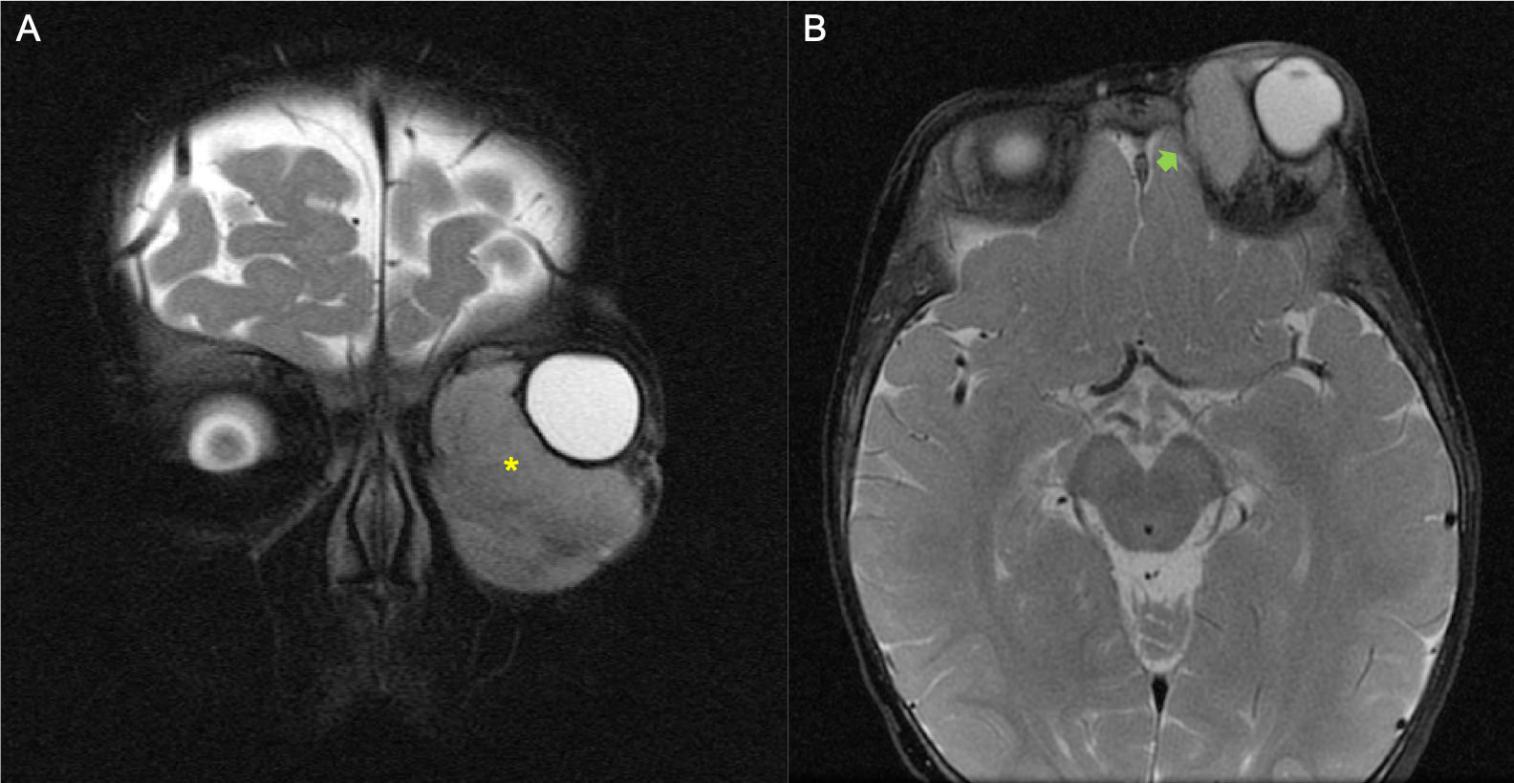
Example of a patient with orbital rhabdomyosarcoma (RMS) and minimal bone erosion (BE). (A, B) MRI images showed left orbital tumor on T2-weighted coronal plane (*yellow asterisk*) (A) and minimal grade of BE on the axial fat-saturated T2-weighted image (*green arrow*) (B).
